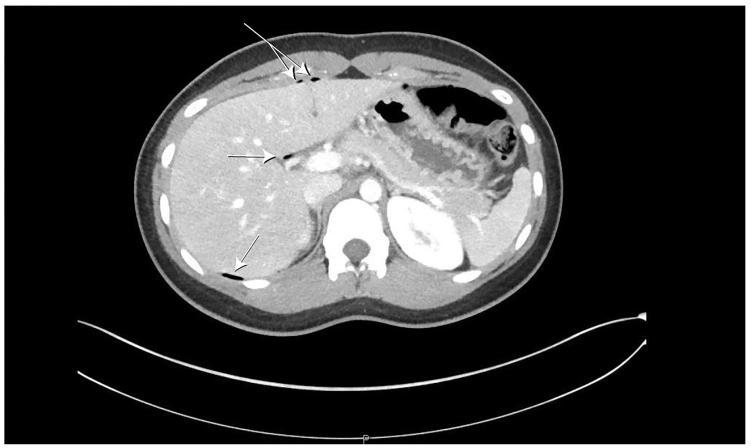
Axial CT image demonstrating free air (arrows) in superior abdomen.
